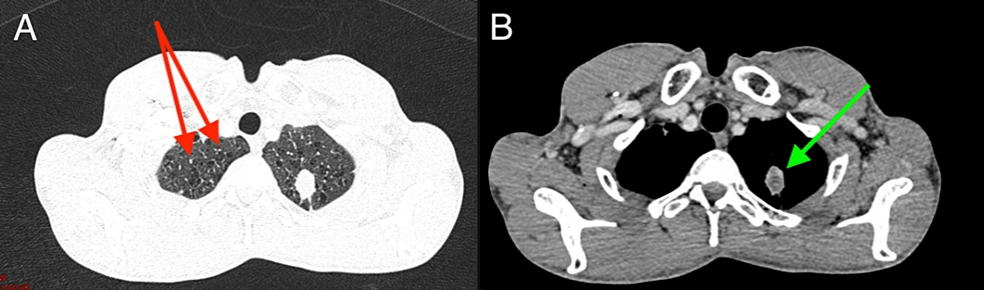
Axial chest CT scan A: lung window; B: mediastinal window demonstrating spiculated parenchymal lung nodules in the left apical region (green arrow), with bilateral pulmonary parenchymal nodules and micronodules (red arrows), with the largest measuring 9 mm in the sub-pleural middle lobar fissure and 8 mm in the sub-pleural right lower lobe.
